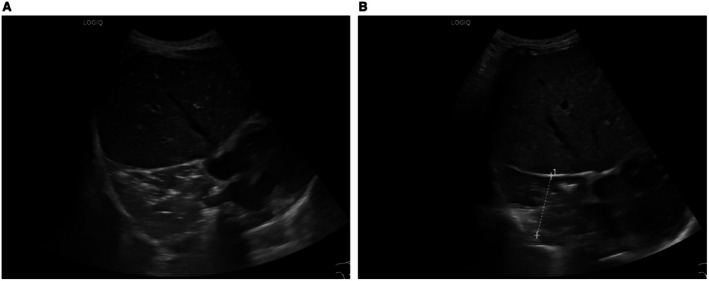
(A) Pleural ultrasound showing atelectasis 1 day post removal (day 5 after aspiration event). (B) Pleural ultrasound showing atelectasis 3 days post removal (day 7 after aspiration event).
